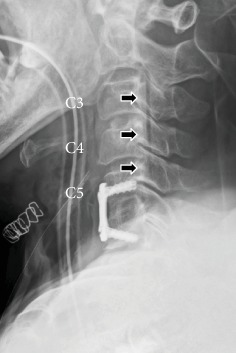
Case 1. Postoperative X-ray (lateral view). A black line (possibly air) was observed posterior to the cervical vertebrae between the bottom of the C2 vertebra and the middle of the C5 vertebra (black arrows).
